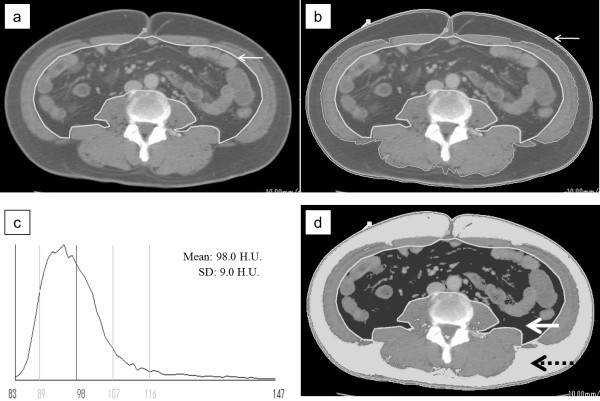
CT images obtained at the navel level demonstrating the method used to determine abdominal fat distribution. (a) The line (arrowed) outlines the intraperitoneal area. (b) The line (arrowed), drawn with a cursor automatically or manually, outlines the subcutaneous fat layer, in which attenuation is measured. (c) A histogram of the CT numbers (in Hounsfield units) in the area outlined in b (mean ± 2 SD). (d) Measurement of visceral fat tissue (solid arrow). The total fat area is represented by the region outlined by the circumference of the abdominal wall. The visceral fat area was subtracted, and the remainder was regarded as the subcutaneous fat area (dotted arrow).
